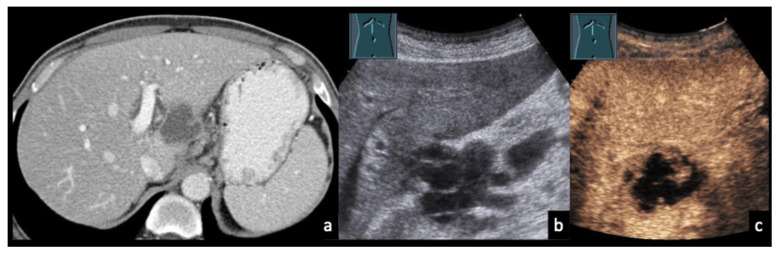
57-year-old male patient with confirmed lymph node tuberculosis in the hepatic hilum ((a), CT). (b) B-mode ultrasound demonstrates multiple partially anechoic lymph nodes. (c) CEUS shows a large non-enhancing area, consistent with extensive necrotic transformation.
